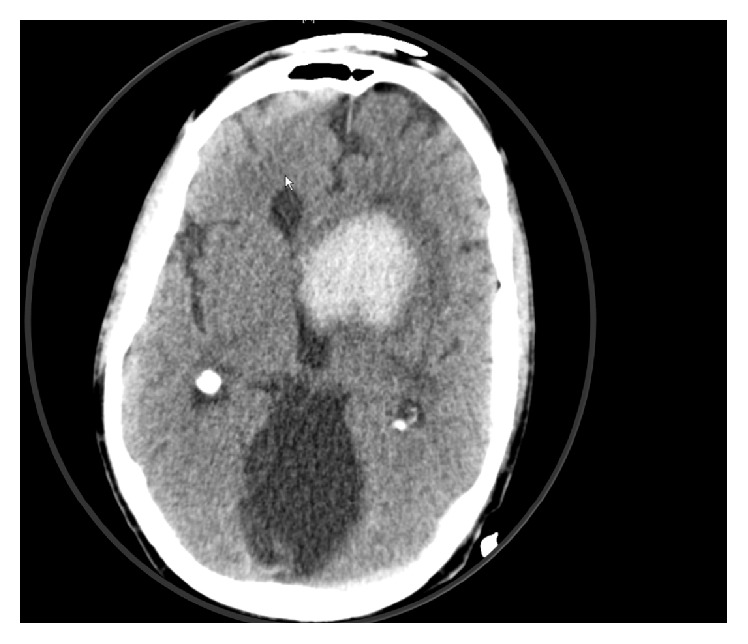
Angio-Head-CT shows a frontobasal left sided intracerebral bleeding with irruption into the ventricles and an enlarged ventricle system.
